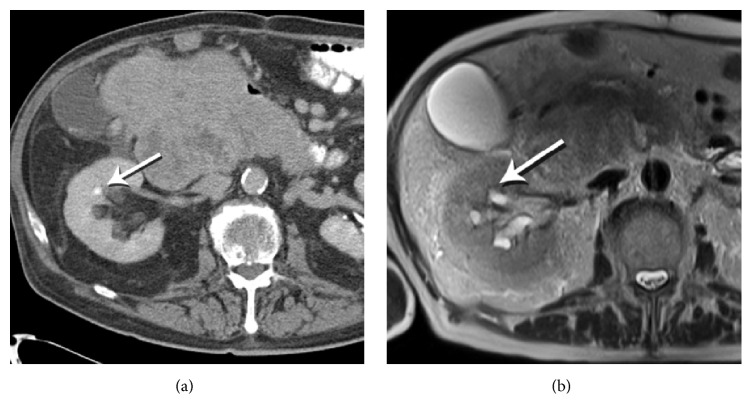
Stone visible on CT, but not on MR. (a) Contrast-enhanced CT performed for tumor staging reveals a stone in a right renal interpolar calyx (arrow). (b) Axial HASTE MRI image fails to detect the stone in the same calyx (arrow).
